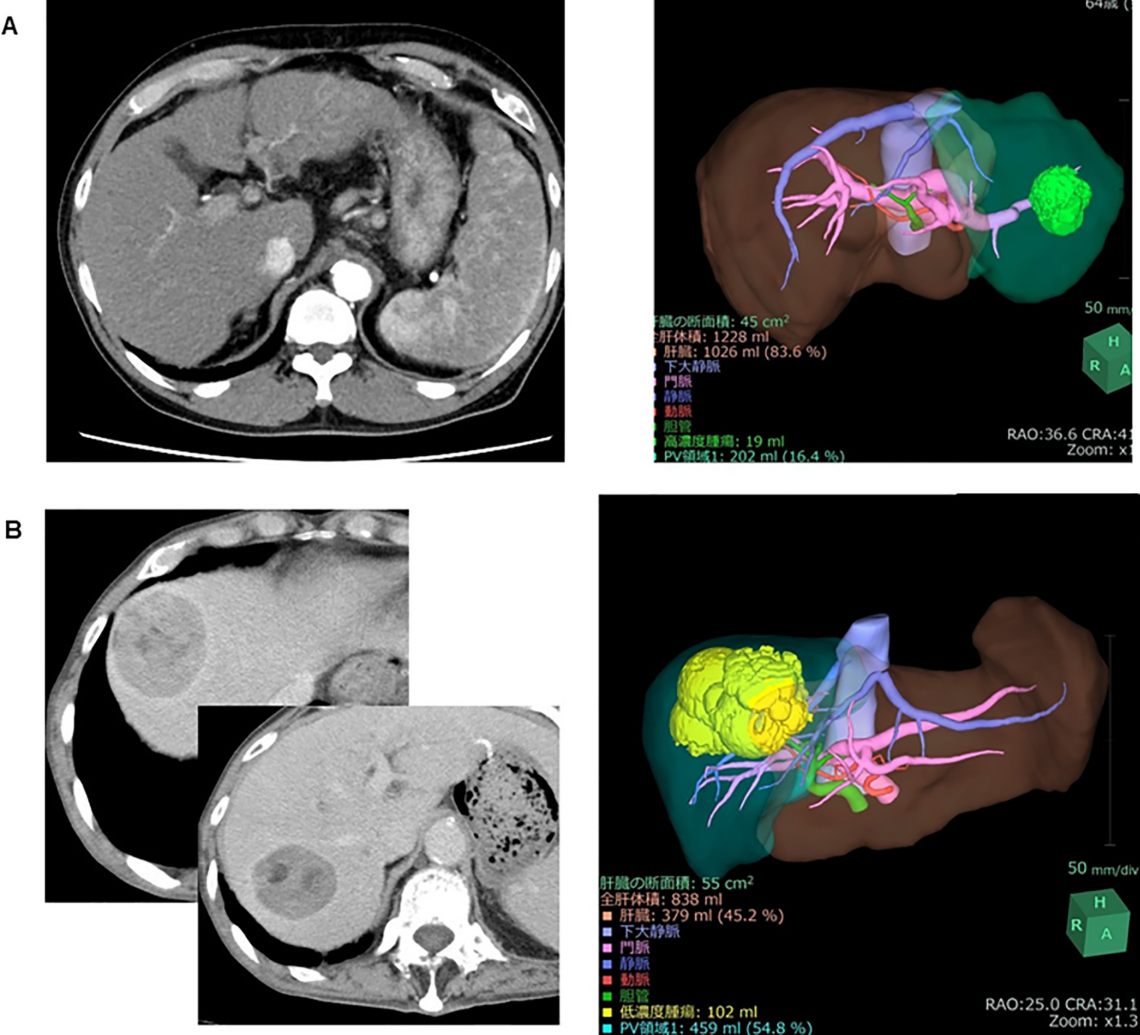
(A) Representative cases in the validation set. Abdominal enhanced computed tomography (CT) shows a very large tumor in the right lobe. Based on 3D volumetry using SYNAPSE VINCENT, the remnant volume after right lobectomy of the liver was 45.2%. (B) Representative cases in the validation set. Abdominal enhanced CT shows the tumor in the lateral segment (arrowhead). On 3D volumetry using SYNAPSE VINCENT, the volume of the lateral segment was 16.4%.
